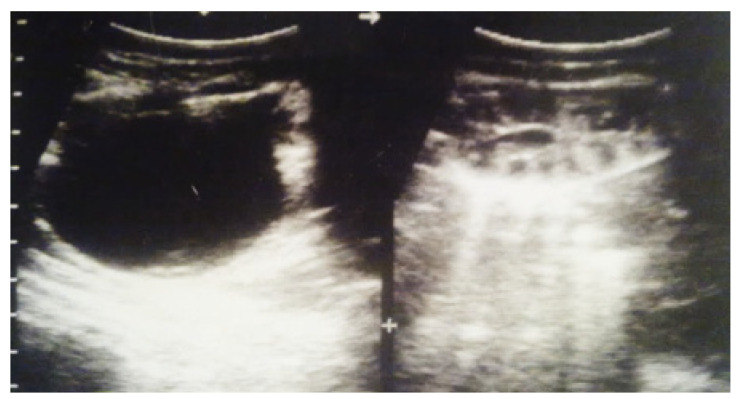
Postnatal urinary ultrasound. Hydronephrosis on the right (newborn 10 days of age).
